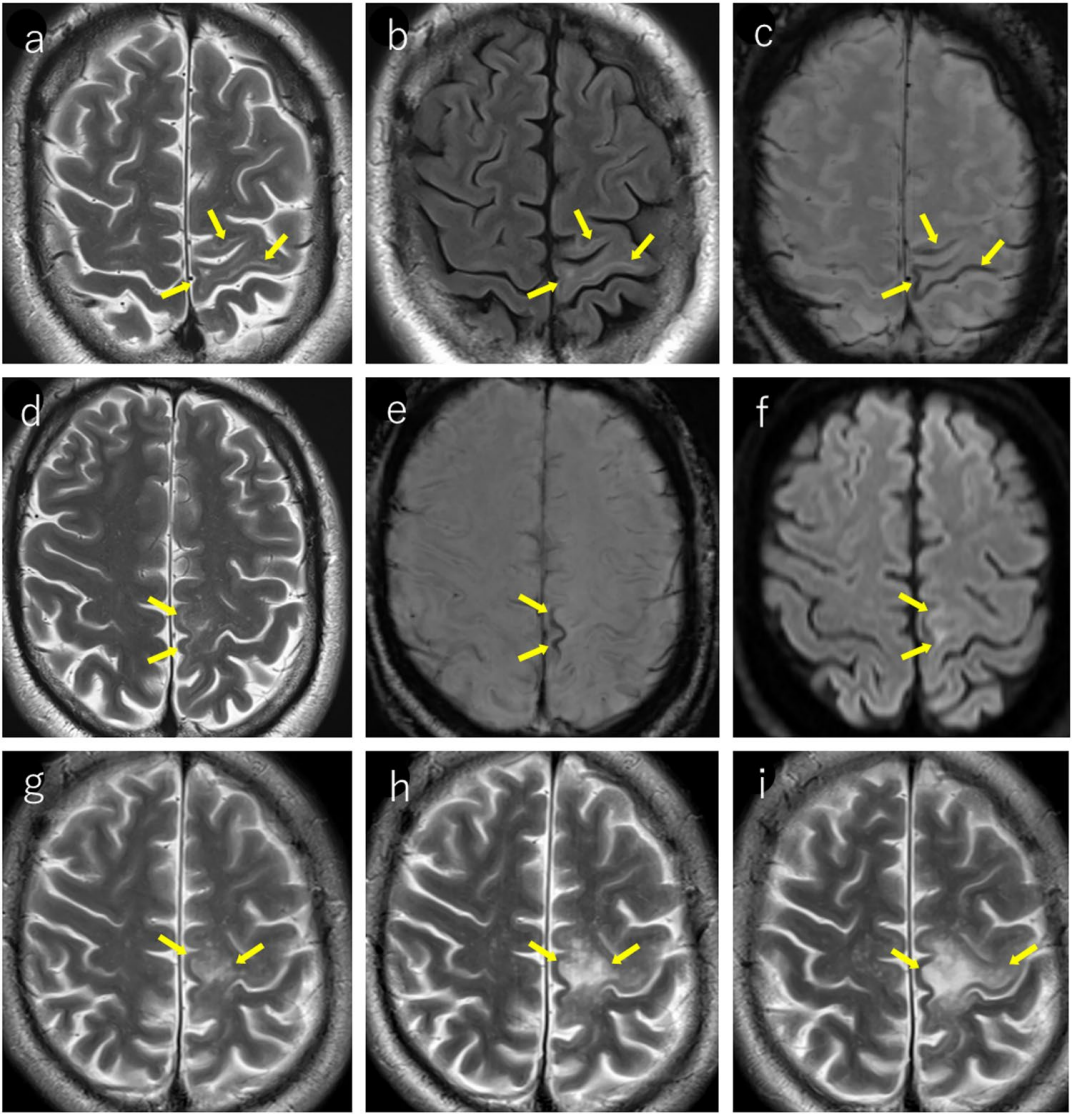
The same patient as in Fig. 2: an HIV-positive man with PML in his 30 s. Initial MRI findings (a–f): a linear T2 hyperintense lesions were observed near the corticomedullary junction, primarily in the left precentral gyrus (yellow arrows). b These lesions appear more conspicuous as hyperintense areas on FLAIR imaging (yellow arrows). c Linear hypointensity is noted on SWI in the cortex adjacent to the lesions (yellow arrows). 5-mm caudal slice findings (d–f): d linear T2 hyperintense lesions present at the cortico-medullary junction of the left precuneus (yellow arrows). e SWI showing linear hypointensity in the deep cortical layers and cortico-medullary junction in parts of the lesion (yellow arrows). f Lesions demonstrating linear hyperintensity on diffusion-weighted imaging (yellow arrows), making them readily identifiable. Follow-up findings (g–i): g 2-month follow-up showing lesion expansion into the subcortical region with a milky way appearance (yellow arrows). h, i Further progression of lesions within the subcortical white matter was observed at the and 4-month follow-up (yellow arrows)
